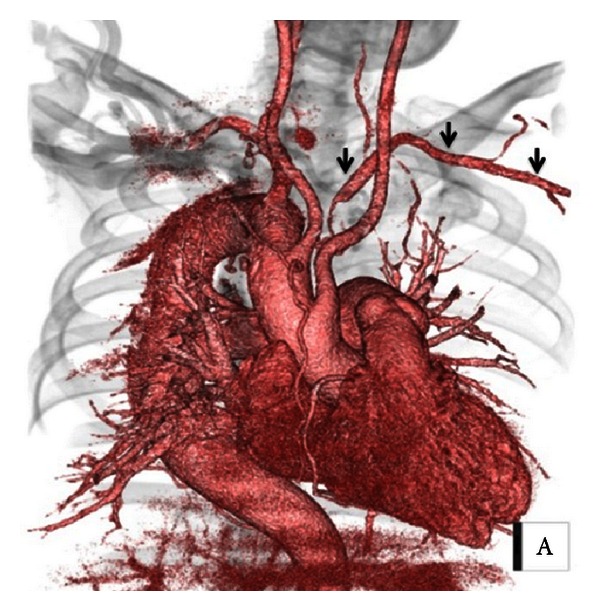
Postoperative CT revealed enhanced ALSA (arrows) clearly without delay, which was supplied with blood from many branches through very narrow and invisible collateral arteries.
